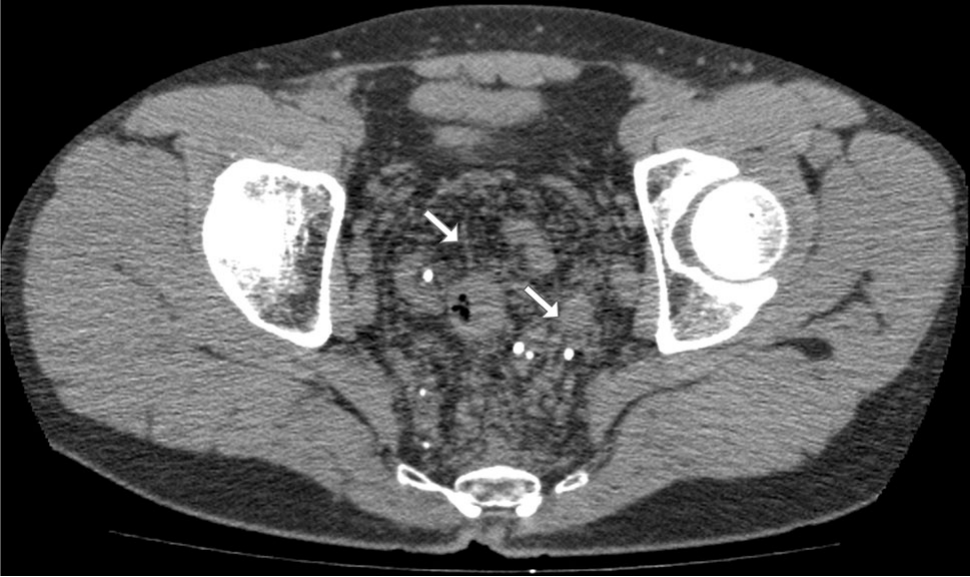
Computed tomography. The mucosal wall from the sigmoid colon to the rectum is thickened and surrounded by fatty tissue enhancement. Typical multiple calcified foci (*arrows*) are also seen
